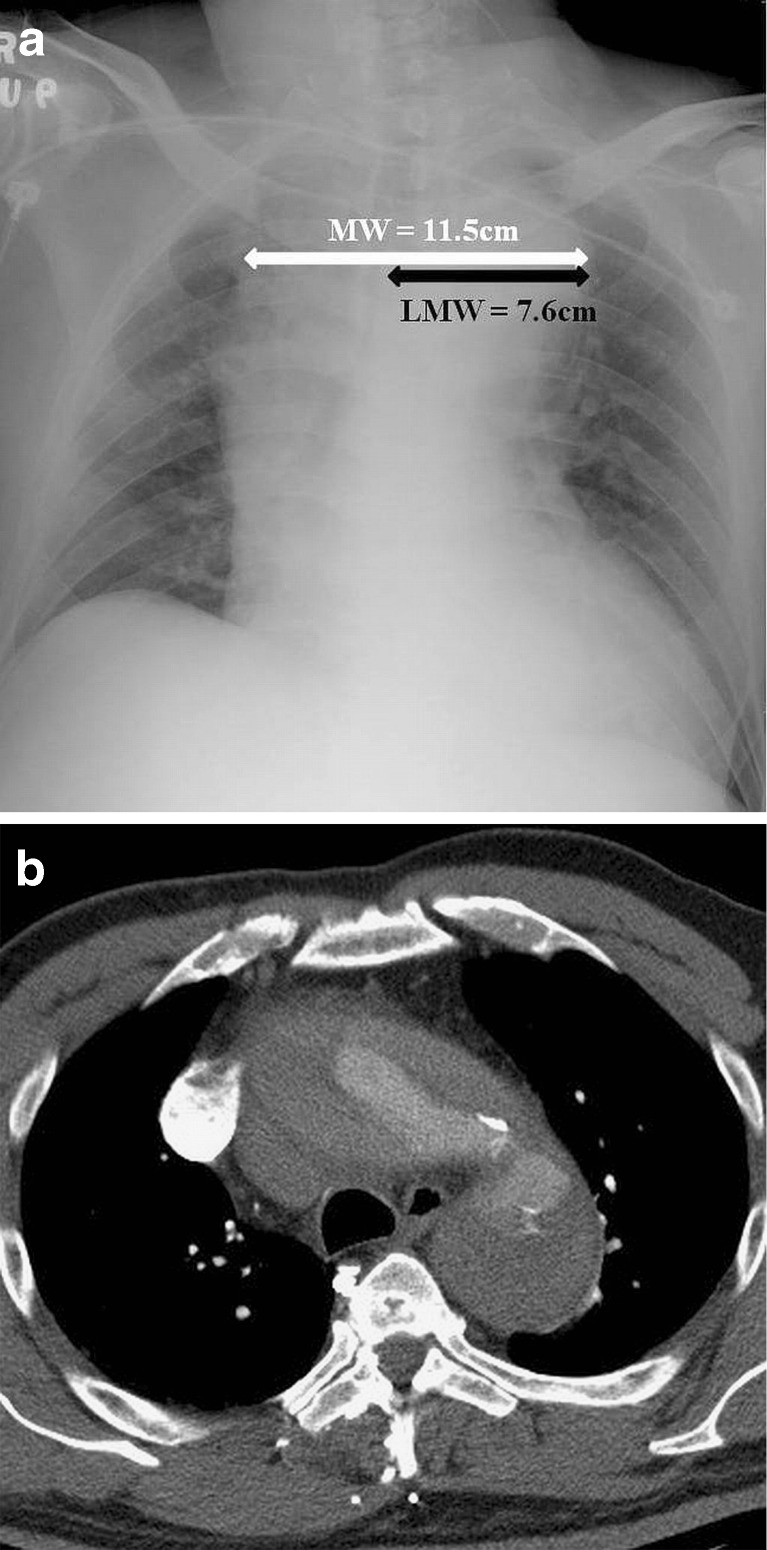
Acute type A aortic dissection in a 46-year-old man. a AP chest radiograph showing marked widening of the mediastinum with MW and LMW measuring 11.5 and 7.6 cm, respectively. b Corresponding selected image of CT aortogram confirms type A aortic dissection
